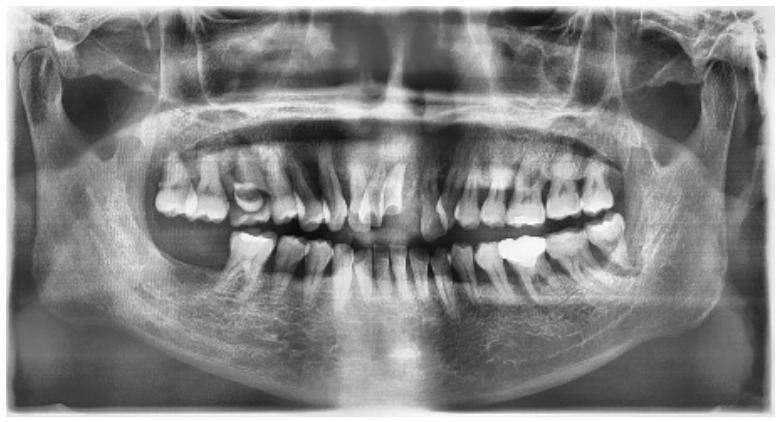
Panoramic radiography at initial visit.
